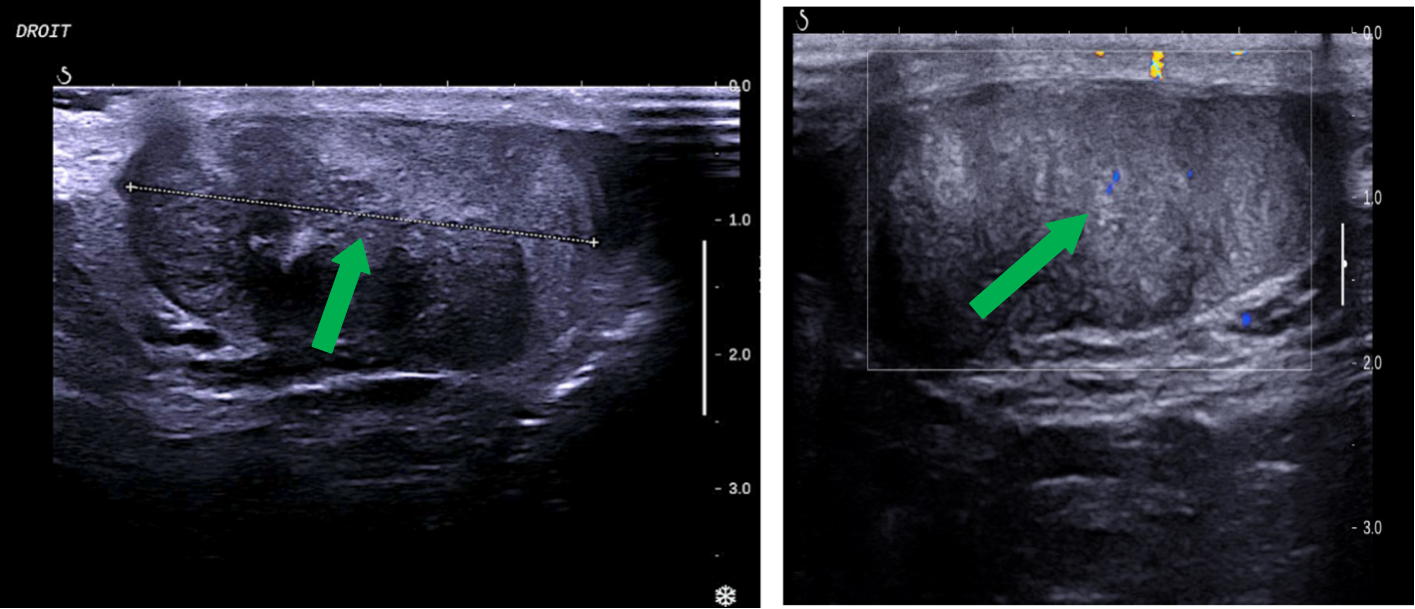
Scrotal ultrasounds of the right testis from second case. Legend. Arrows indicate Testicular Adrenal Rest Tumors (TART)
